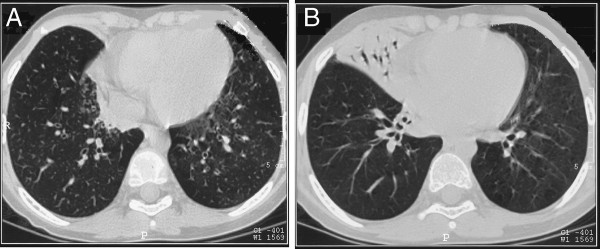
Chest HRCT: bronchiectasis in the left and right lower lobes (A) and a consolidation area in the middle lobe (B) may be observed.
